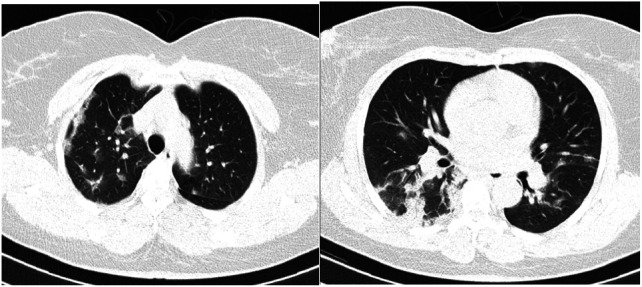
50 year old male with three-day history of cough and fever. CT scan of the chest showing bilateral, peripheral and subpleural consolidation (Negative initial PCR testing. Negative repeat PCR within 3 days)
